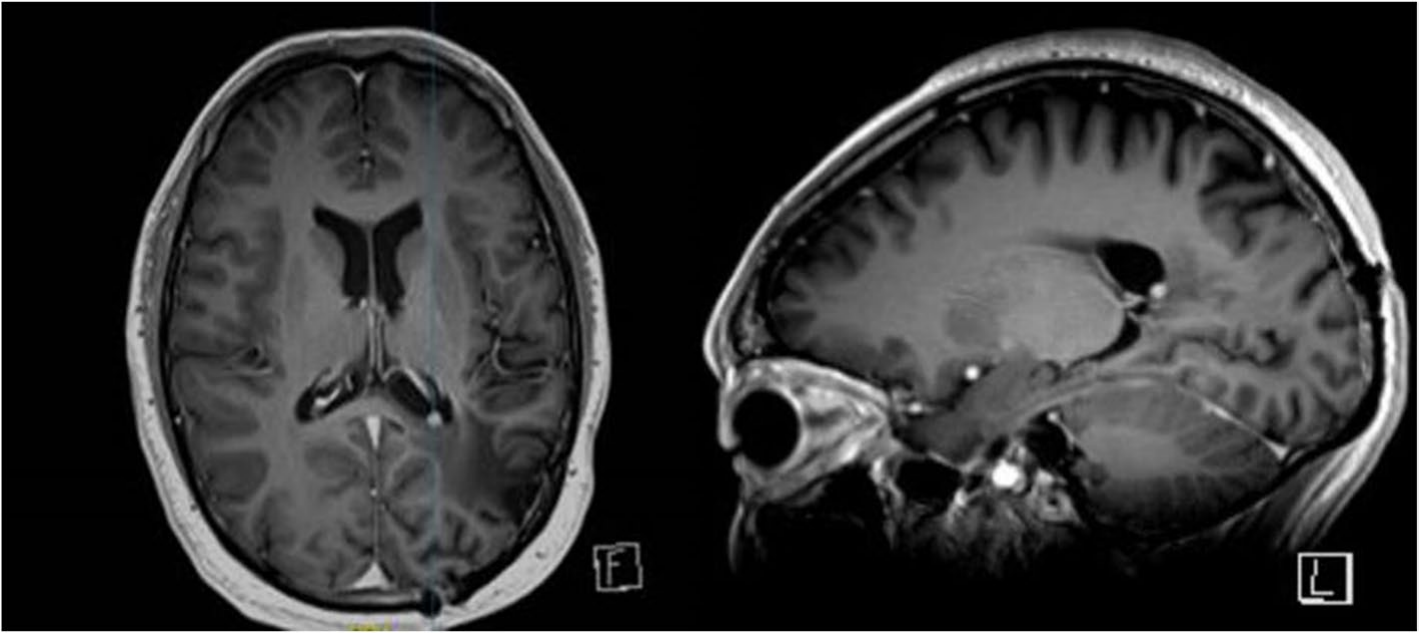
Surveillance MRI 7 years later showing a 4 mm homogenous contrast enhancing lesion within the left ventricular trigone, without restricted diffusion
